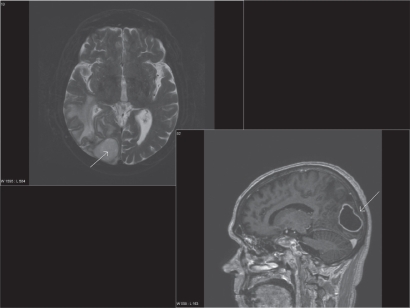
Axial T2 weighted and sagittal T1 weighted magnetic resonance image (MRI) after iv contrast administration: brain metastasis vs. abscess?
